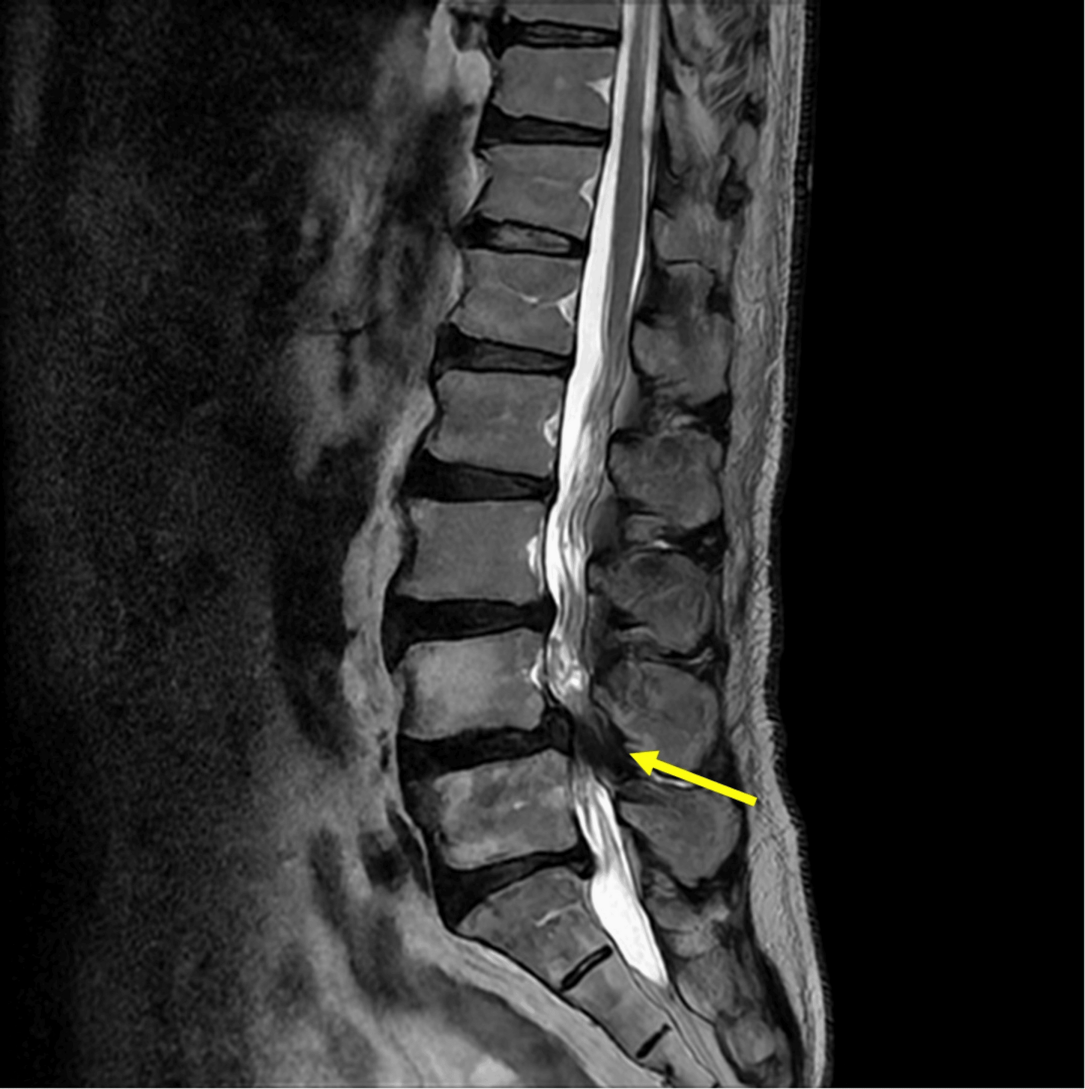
Results of the lumbar MRI. Lumbar MRI showing disc bulging at the L3/4, L4/5, and L5/S1 levels, with prominent stenosis at L4/5 (arrow). MRI: magnetic resonance imaging
